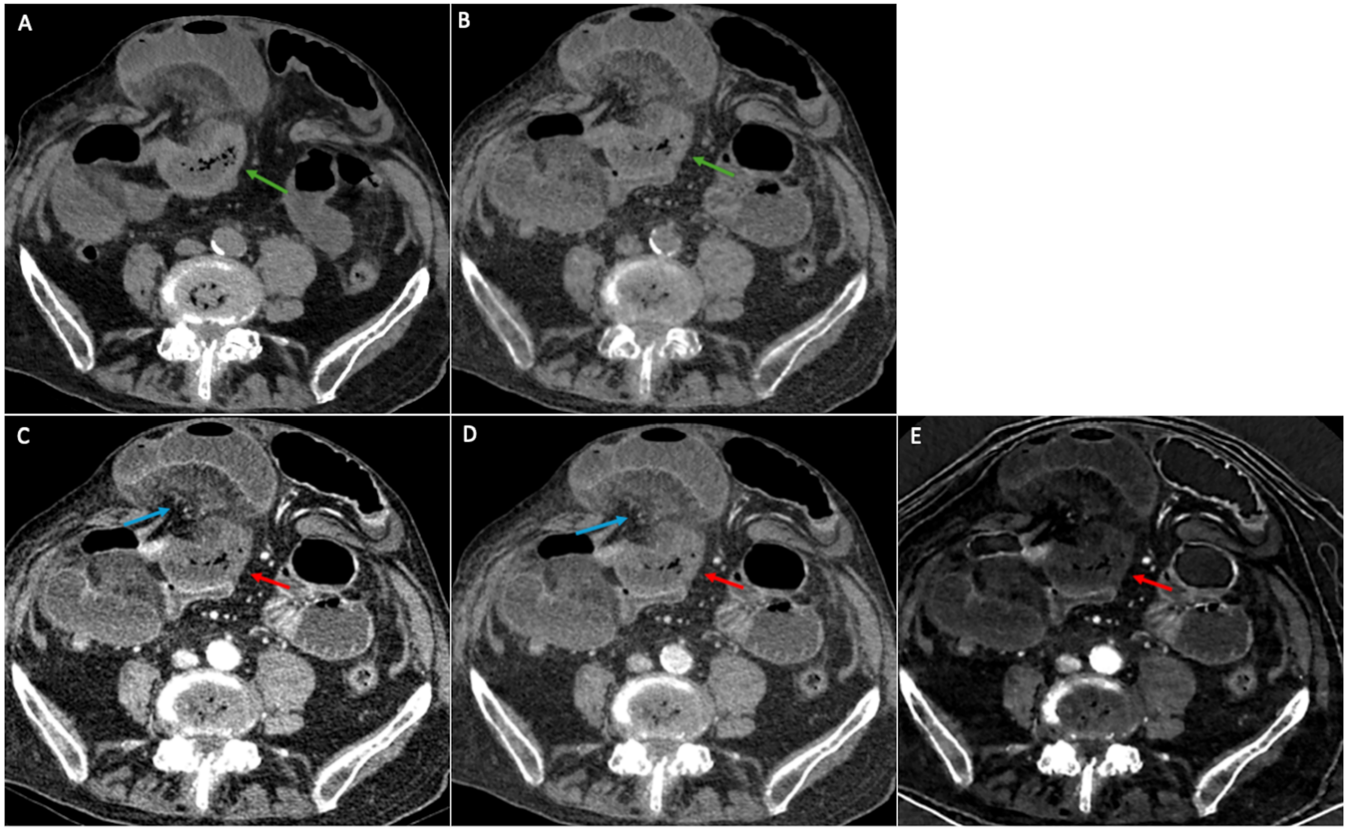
CT images in a 94-year-old woman with small-bowel ischemia secondary to incarceration within an umbilical eventration. The patient was treated by surgery and underwent bowel resection. Axial (A) TUE and (B) VUE images show increased bowel wall attenuation of the involved loop (green arrows). Axial monoenergetic images at (C) 50 keV and (D) 77 keV show a reduced bowel wall enhancement of the involved loop (red arrows) and presence of mesenteric haziness (blue arrows). E Iodine image also shows a reduced mural iodine uptake in the involved loop. The mural iodine concentration of the involved loop was 1.3 mg/mL
